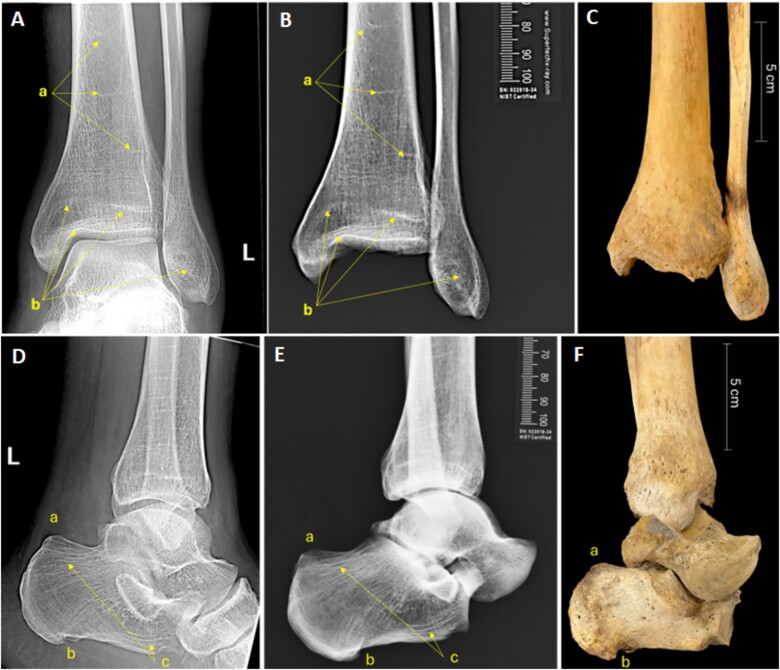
Antemortem radiographs (A, D), postmortem radiographs (B, E), and postmortem photographs (C, F) of the left distal lower limb including the tibia and fibula (A, B, C) and the calcaneus and talus (D, E, F). Harris lines in the distal tibia (a in A and B) as well as the overall trabecular pattern of the tibia and fibula (b in A and B). Overall trabecular pattern of the left calcaneus (a, b, c in D and E).
